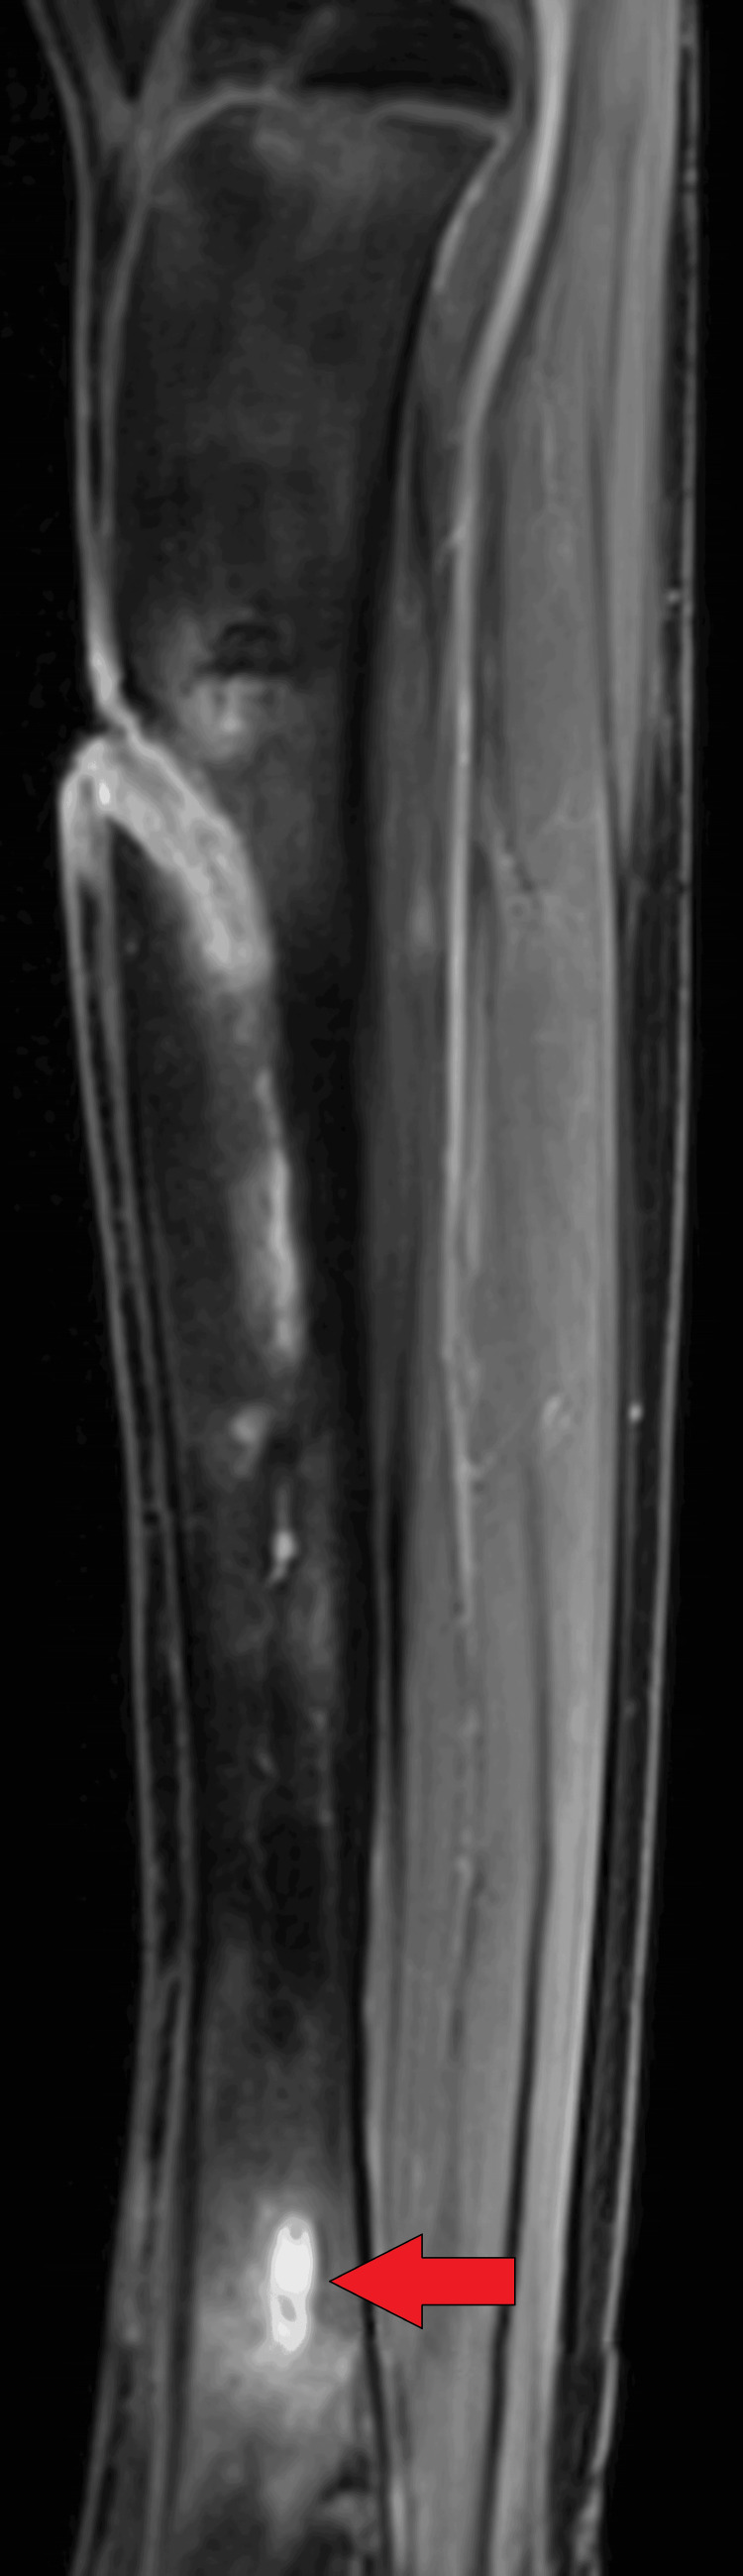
Sagittal T1WI contrast image showing tiny focal areas of post-contrast enhancement involving the lower tibial diaphysis suggestive of intraosseous abscess T1W1: T1-weighted contrast imaging
